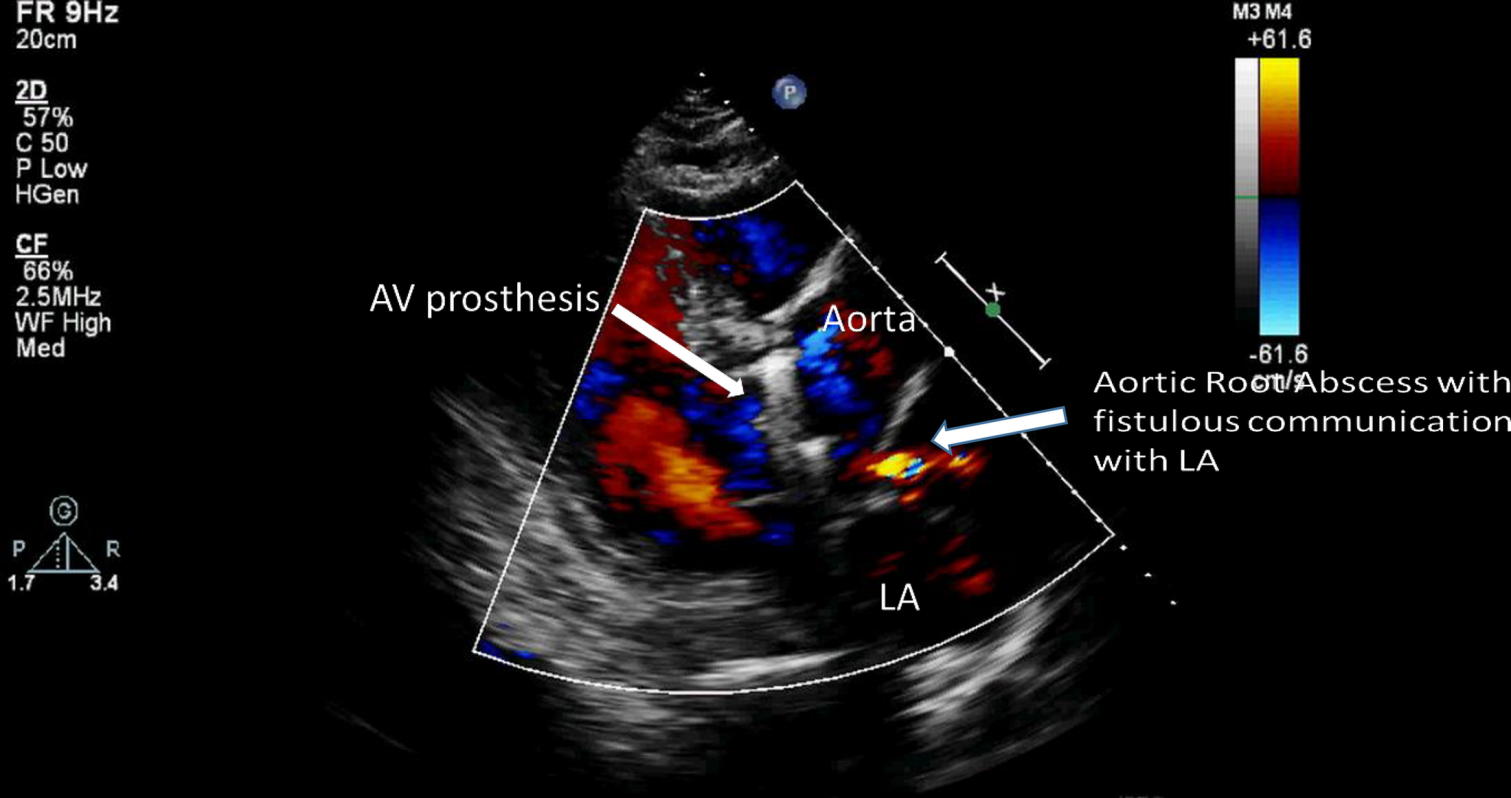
Transthoracic echocardiography showing a metallic aortic prosthesis with an aortic root abscess complicated by a fistula to the left atrium.
